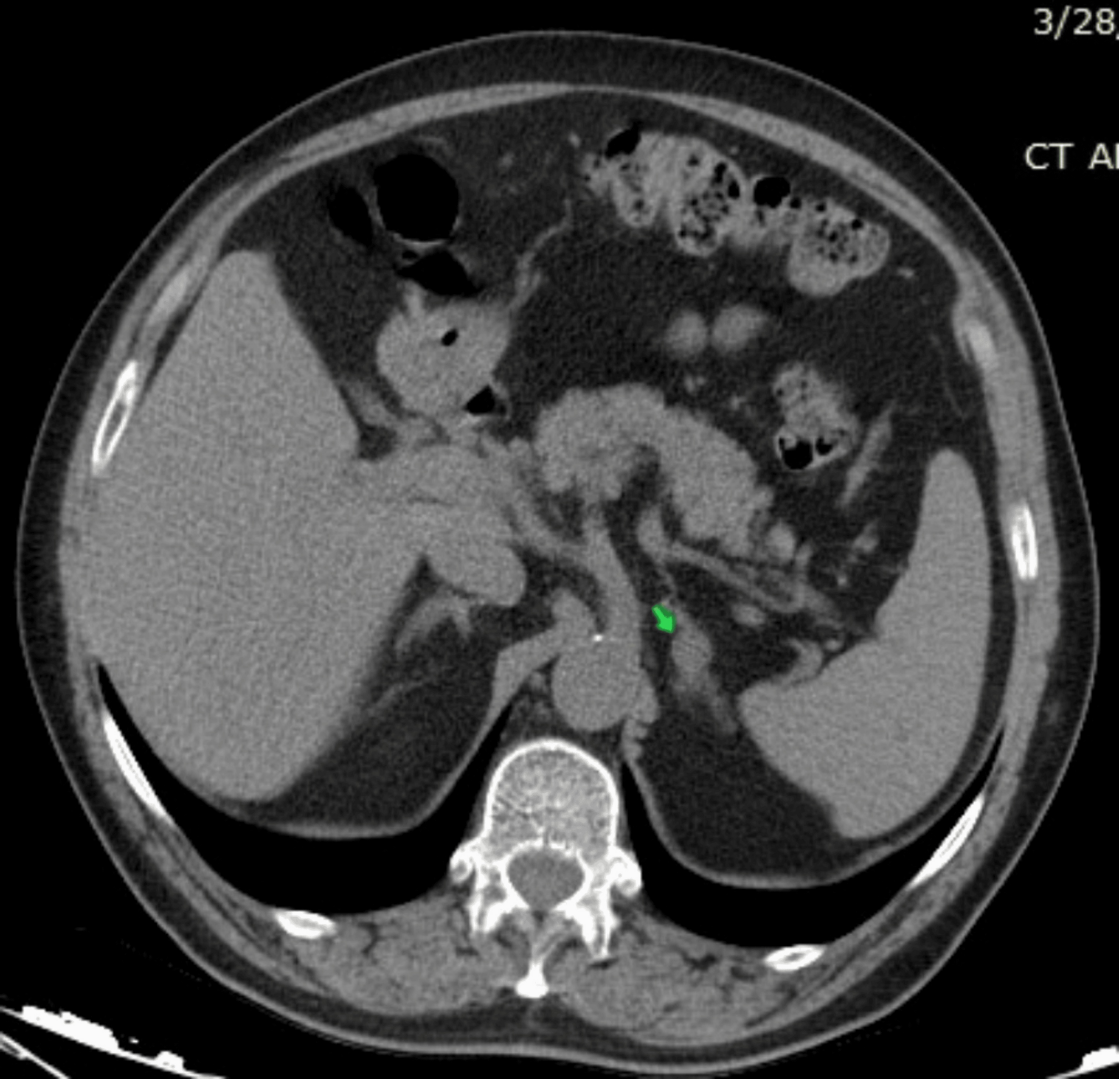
Computed tomography scan of the abdomen The arrow indicates the hypodense nodular formation in the left adrenal gland.
